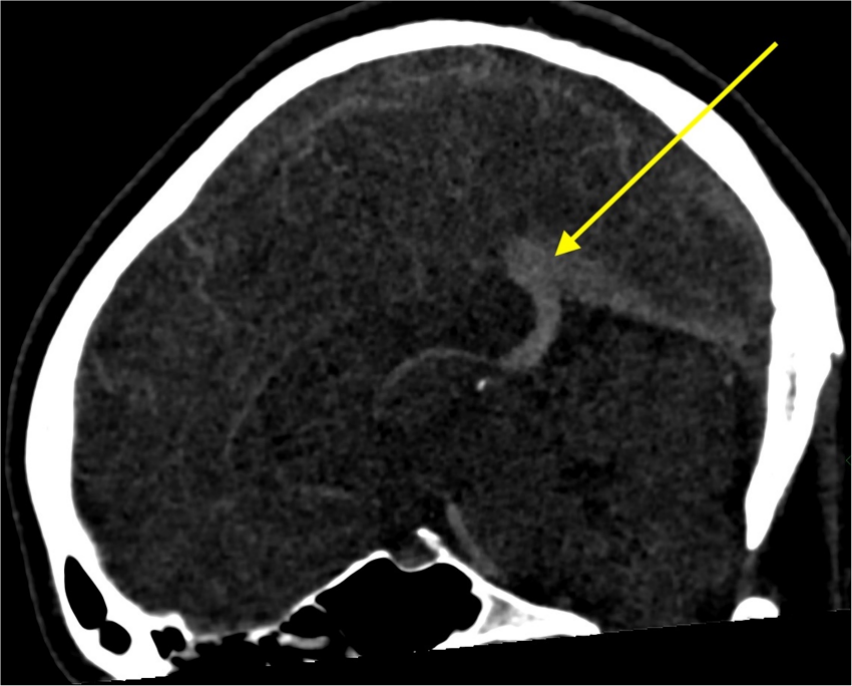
CT venography showing venous thrombosis.
